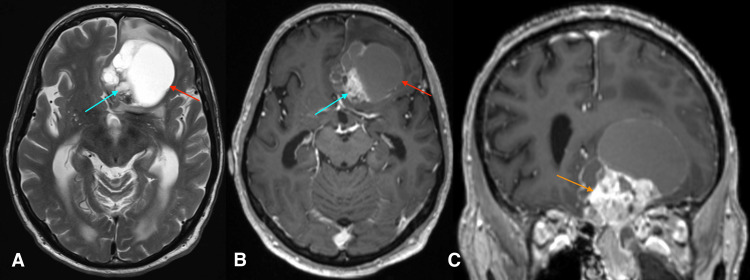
Magnetic resonance imaging of the brain T2-weighted in the axial plane (A), T1 post-gadolinium contrast in the axial (B) and coronal planes (C) T2-weighted imaging demonstrates a hyperintense cystic mass (red arrow) with a solid mural component within the medial left frontal lobe (blue arrow). Post-gadolinium imaging demonstrates a mixed cystic (red arrow) and solid mass with enhancement of the solid components along the posteromedial wall (blue arrow). The enhancing extra-axial soft tissue extends through the cribriform plate (orange arrow), severely distorting the adjacent frontal lobe.
